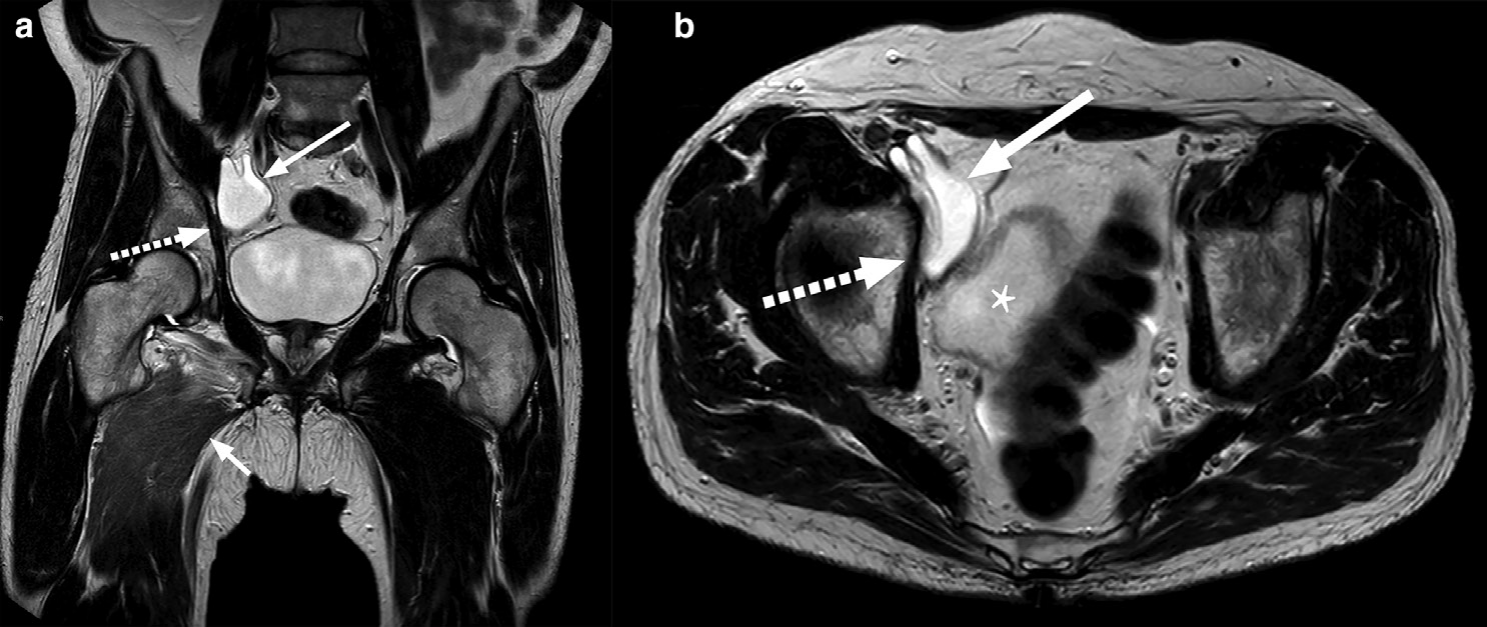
MRI T2 TSE in coronal (a) and transverse (b) views, demonstrate the intra-abdominal reservoir (arrow) compressed between the right pelvic side-wall (dashed arrow) and bladder (asterisk, b). This is the presumed site of the obturator nerve compression, immediately proximal to its entrance into the obturator foramen. We can also observe high signal oedema in the right adductor musculature (short arrow, a) in keeping with denervation oedema secondary to compression of the obturator nerve. TSE, turbo spin echo.
